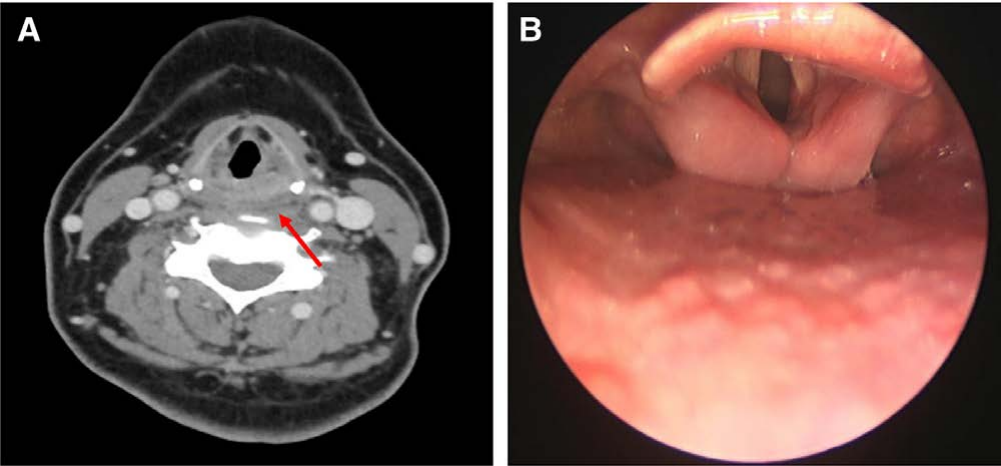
CT scan and laryngoscopic findings after treatment. (A). CT shows hematoma (arrow) resorption at the level of the arytenoid cartilage. (B). The obstruction in the glottic region has disappeared. CT = computed tomography.
